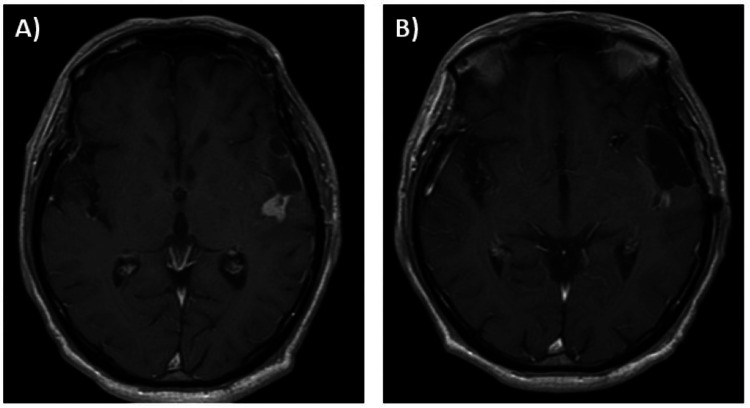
MRI brain findings (Case 2) (A) One months after steroid therapy, contrast lesion had shrunk; (B) Two months after steroid therapy, the contrast lesion had disappeared.
